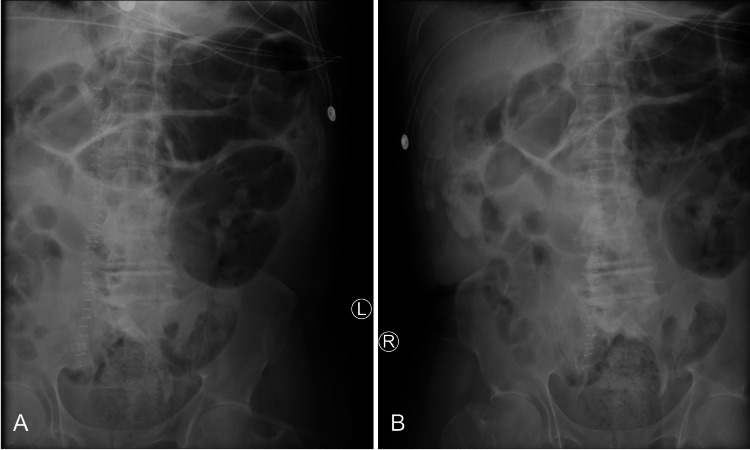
Kidney, Ureter, and Bladde (KUB) Plain Radiograph Kidney, Ureter, and Bladder X-Ray of the left and right abdomen (A and B). Multiple loops of prominent gas-filled small bowel without evidence of high-grade obstruction. Residual changes and material at the right abdomen and left lower quadrant. Nonspecific but nonobstructive bowel gas pattern with postoperative changes.
